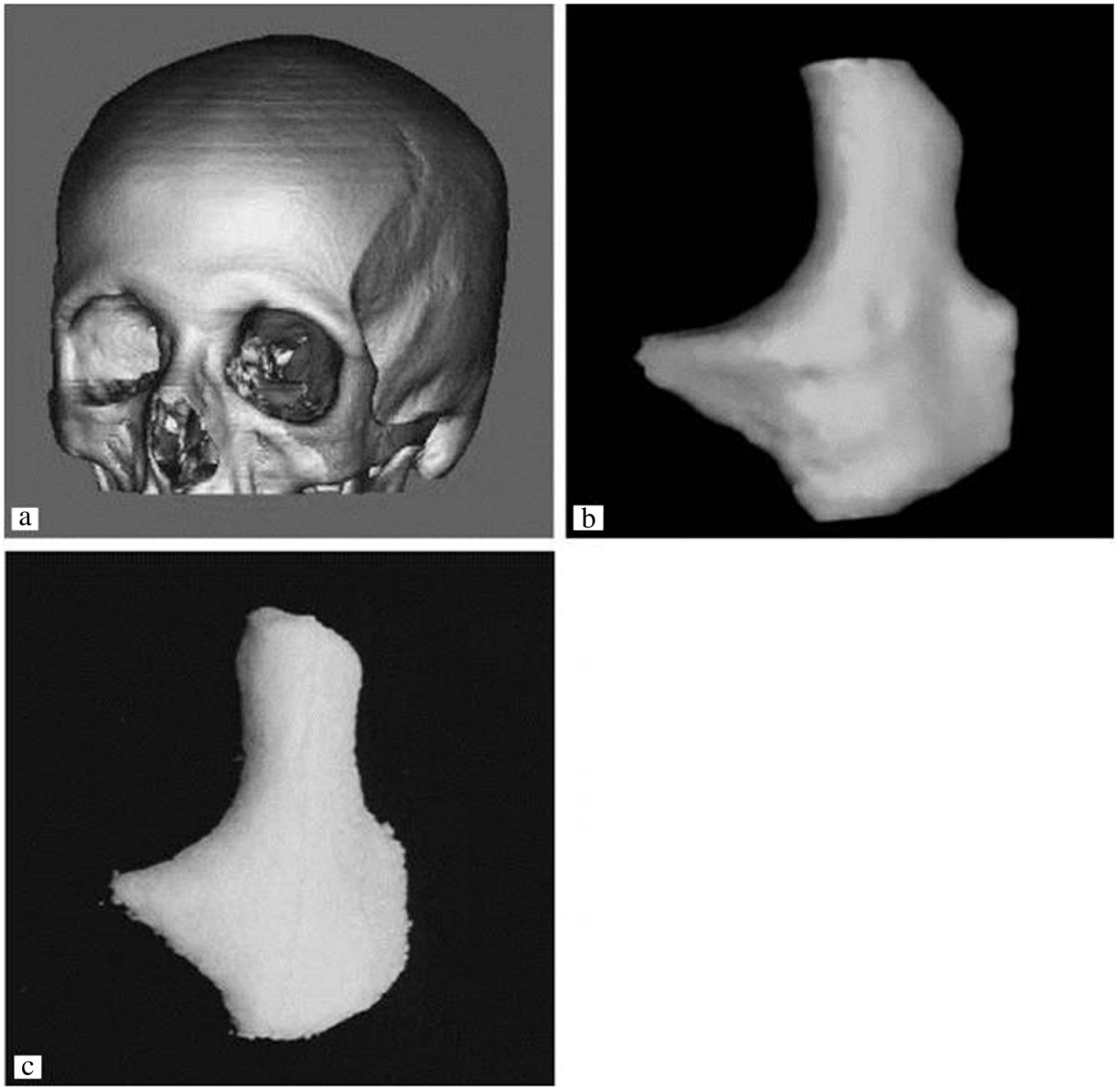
3D printed scaffolds can be patient-specific. A zygoma was generated from CT 2D images (a,b) and zygoma-shaped scaffold was produced from indirect 3DP (c). Reproduced with permission from [17].
